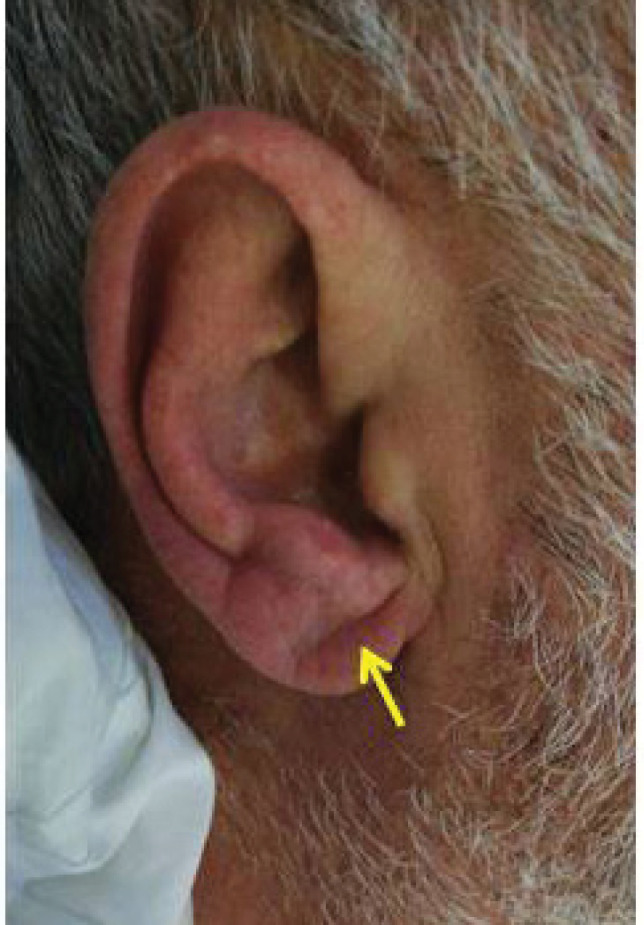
Hemogram, troponin, lipid profile, and other biochemical parameters were analyzed from blood samples taken during admission. Fasting plasma glucose levels ≥126 mg/dL or plasma glucose levels ≥200 mg/dL at any time plus diabetic symptoms or 2^nd^-hour plasma glucose levels ≥200 mg/dL in oral glucose tolerance test or HbA1C levels ≥6.5 were used to define diabetes mellitus (DM). Patients were evaluated with echocardiography by experienced echocardiographers according to the recommendations of the European Association of Cardiovascular Imaging. Biplane Simpson’s method was used for determining the left ventricular ejection fraction (LVEF).^13^
